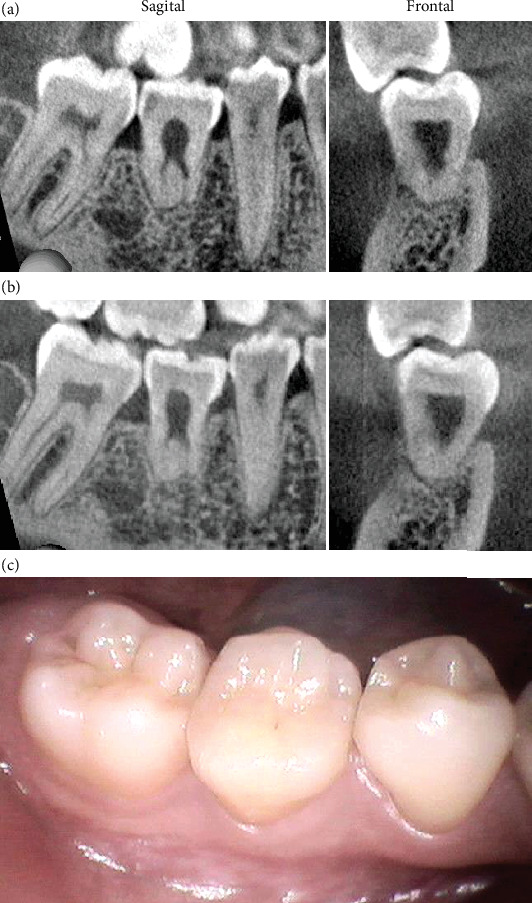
(a) CBCT appearance taken at 9 years after the transplantation. (b) CBCT appearance taken at 11 years after the transplantation. (c) A clinical picture of the transplanted tooth at 11 years after the transplantation.
